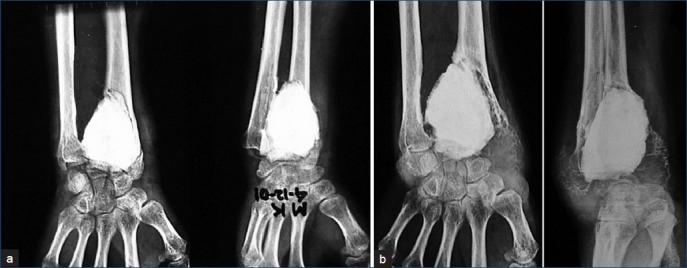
X-rays (anteroposterior and oblique views) of wrist joint showing (a) Two-weeks postoperative X-ray showing bone cementing after curettage. (b) Recurrence after bone cementing at 12 months
